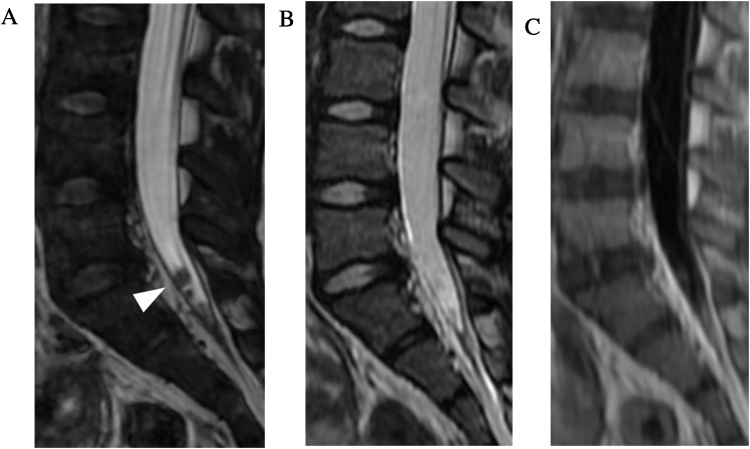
Leptomeningeal drop metastases. 7-year-old girl with history of diffuse intrinsic pontine glioma. Sagittal FIESTA-C MRI image (a) through the caudal thecal sac demonstrates nodular metastatic deposits (arrowhead), which are poorly seen on sagittal T2-weighted FSE (b) and sagittal contrast enhanced MRI images (c) (slice thickness, 3 mm).
